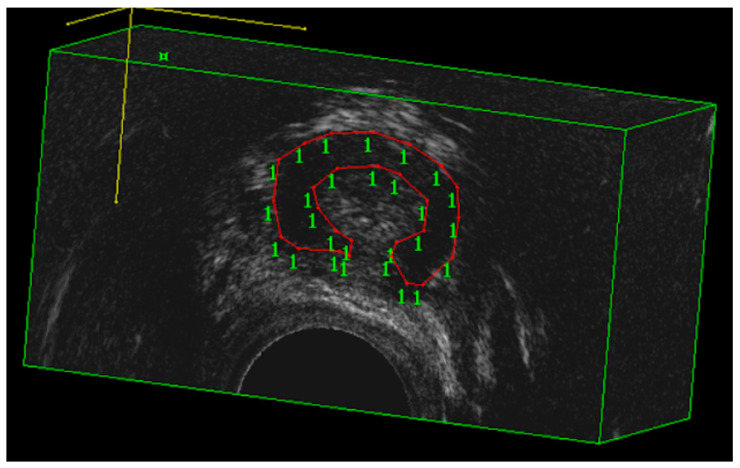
Volumetric assessment of the urethral rhabdosphincter (RS) using a BK Flex Focus 400 sonographic machine equipped with a 9052 transducer by vaginal approach.
